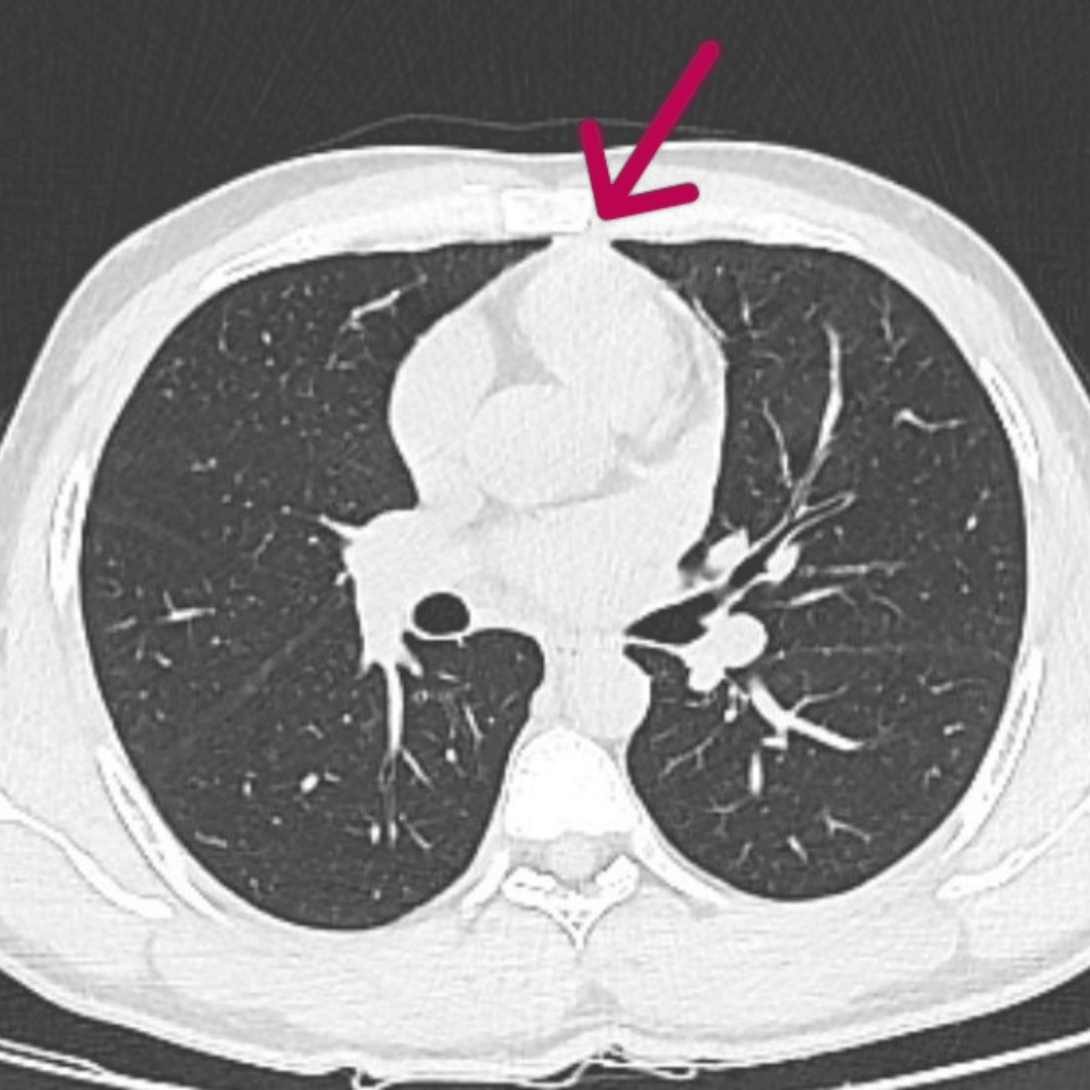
Repeat chest CT showing resolution of pneumomediastinum (taken August 23, 2022).
